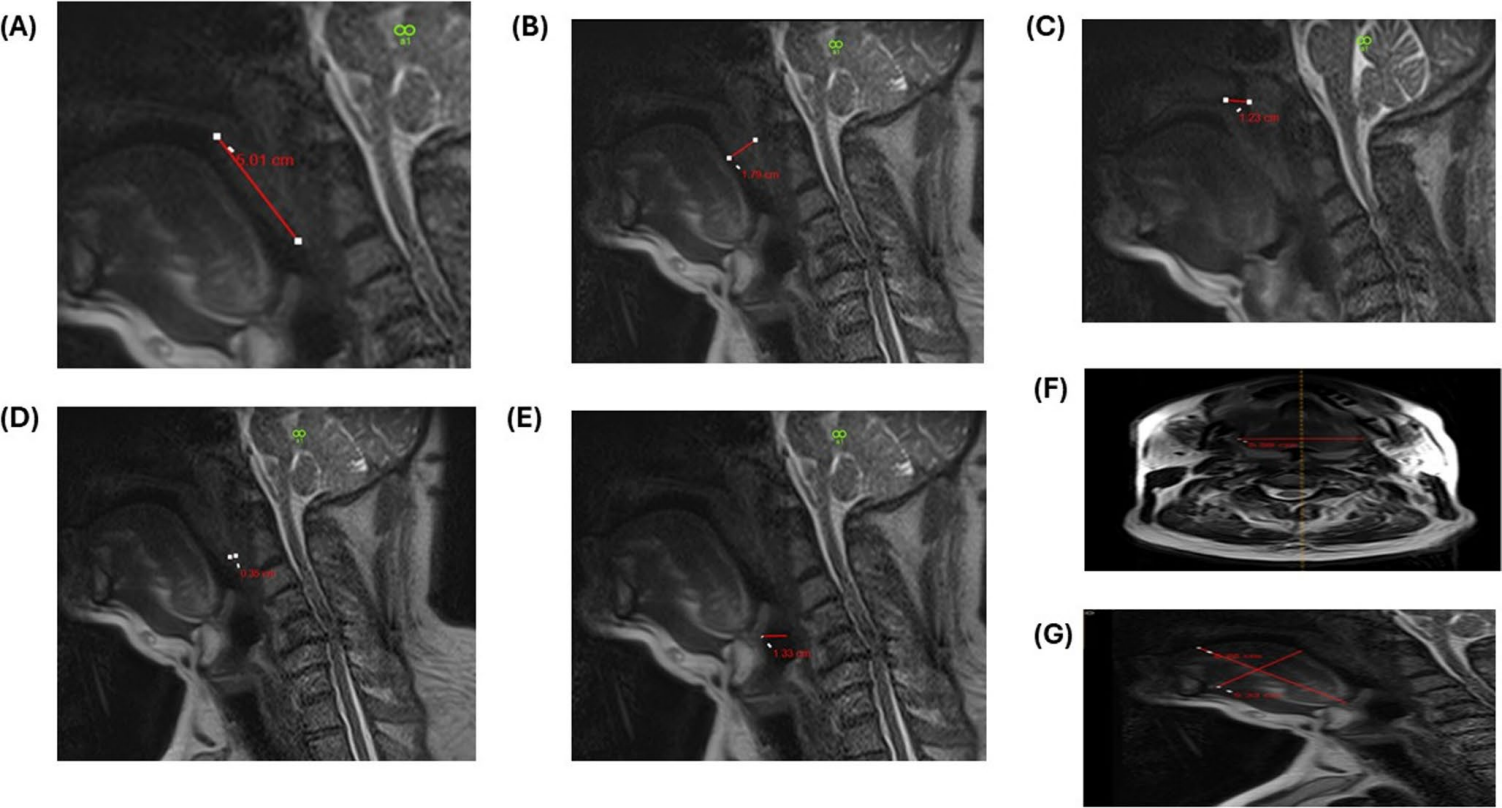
Pre-operative MRI images for assessment of the same patient showing (A) a maximum length of the soft palate (MLSP) of 50.1 mm, (B) a maximum thickness of the Soft palate (MTSP) of 17.9 mm, (C) Posterior nasal airway space (PNAS) of 12.3 mm, (D) Occlusal posterior airway space (OPAS) of 3.5 mm, (E) mandibular posterior airway space (MPAS) of 13.3 mm, and (F) coronal tongue dimensions of 5.59 mm and (G) axial tongue dimensions of 8.76 × 5.33 mm for an estimated tongue volume of 137mL. MRI – Magnetic Resonance Imaging
